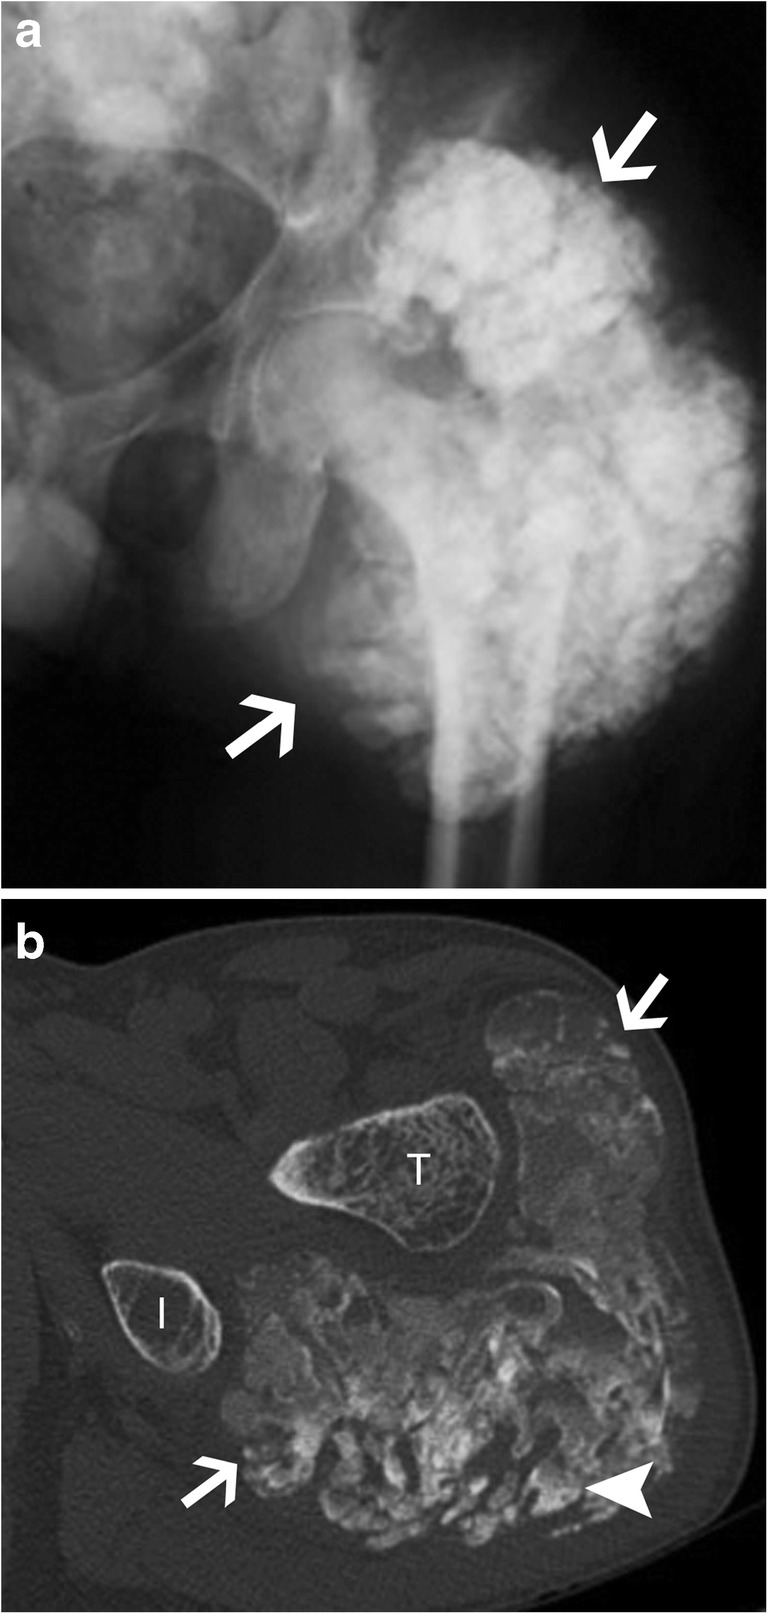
Renal insufficiency with tumoural calcinosis. (a) Anteroposterior radiograph of the pelvis and (b) axial CT image of the left hip show tumoural calcinosis (arrow) around the left hip with a multiloculated appearance in a clinical context of chronic renal failure. Note fluid-fluid level with denser material layering more posteriorly (arrowhead in b). I: ischium, T: greater trochanter
